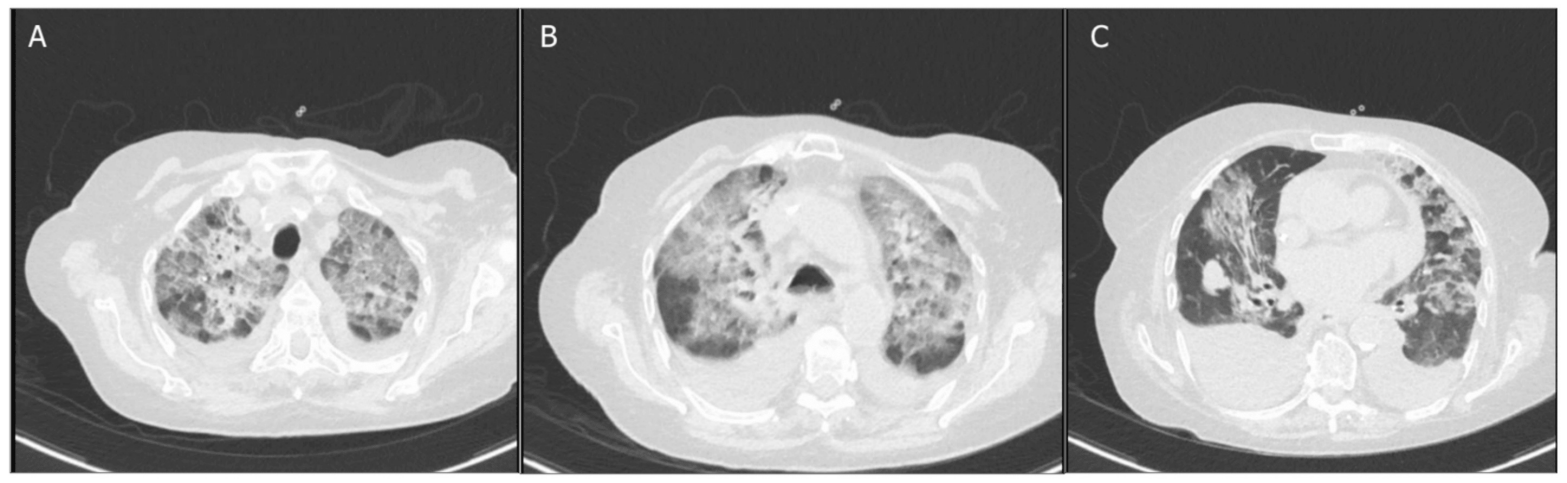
(A-C) Chest computed tomography images showing bilateral pleural effusion and areas with ground-glass opacities and a crazy-paving pattern.
